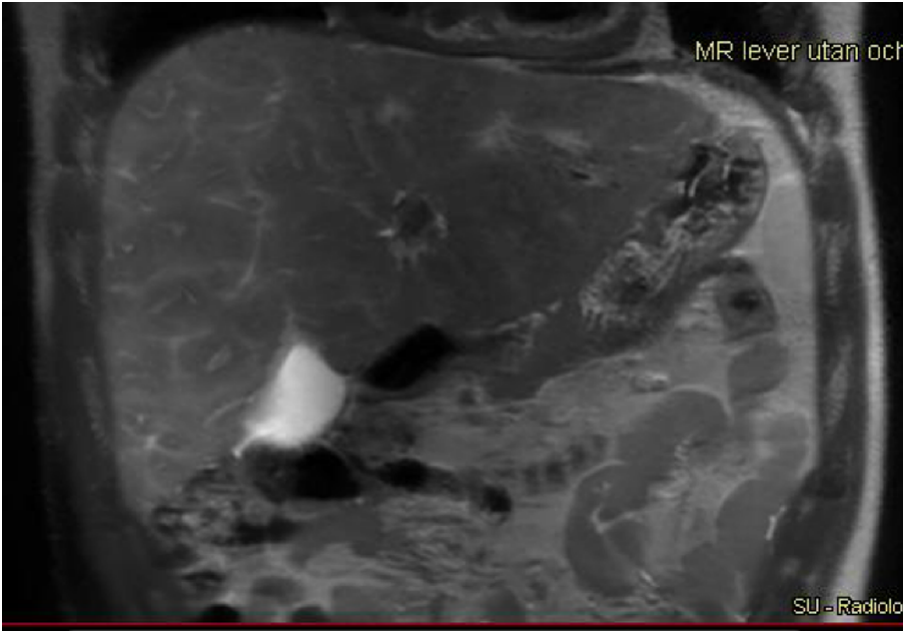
A 17-year-old boy with hypoplastic left heart complex operated with a Fontan circulation. Abdominal MRI, a coronal T2-weighted image, reveals the typical heterogenous distribution of the liver disease with irregular signal, perisinusoidal edema, in the right liver lobe with a more homogeneous signal in the left lobe.
